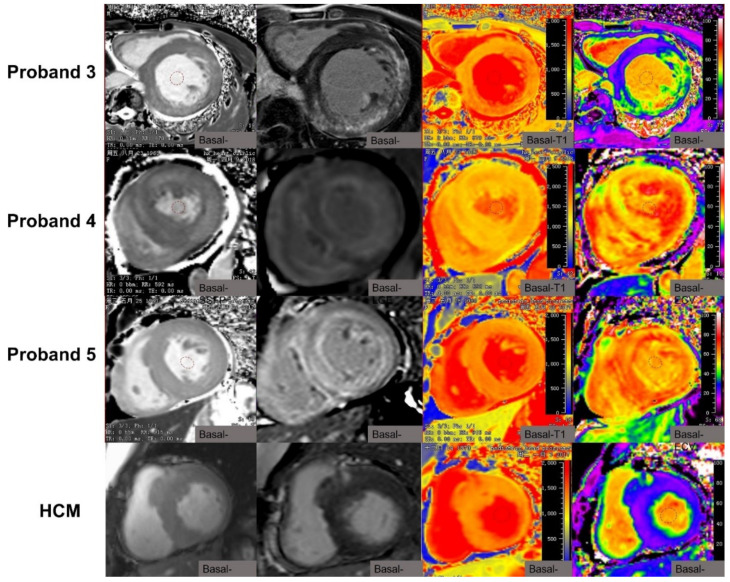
The characteristics of cardiac MRI in the proband 3 patient with *PSKAG2* syndrome, the proband 4 patient with senile systemic amyloidosis, and the proband 5 patient with *TTR*, and a HCM patient with the typical HCM LGE pattern.
